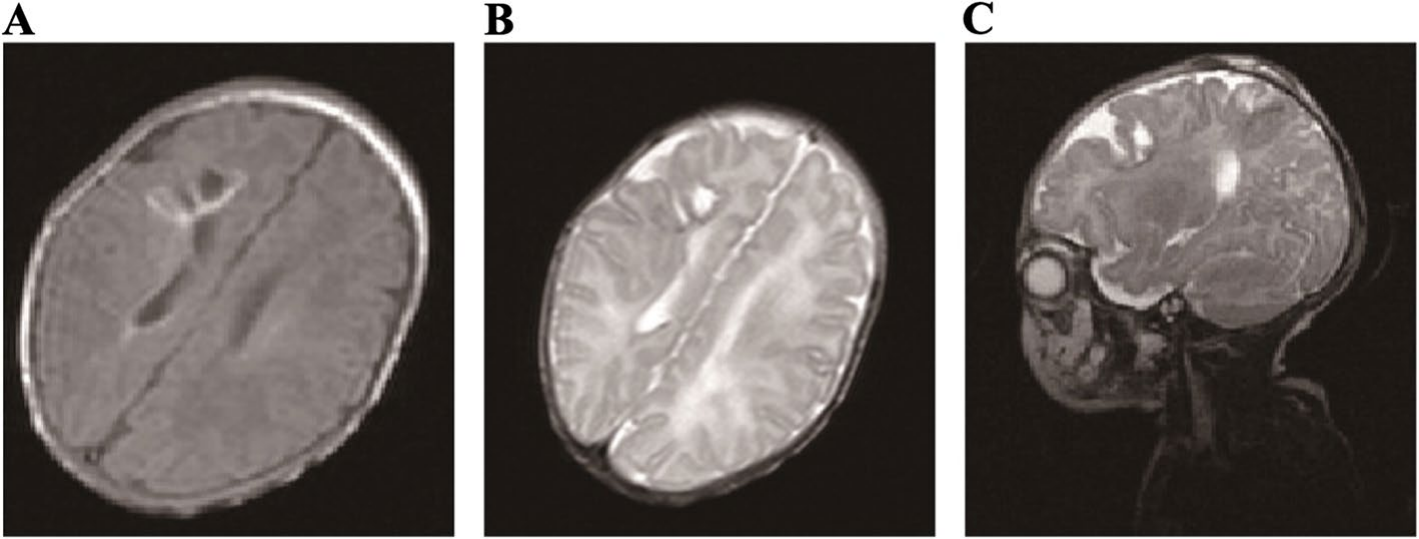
Nearly complete resolution of the abscesses with little hemorrhage. A, B, and C showed the T1-weighted MRI image and T2-weighted MRI image respectively
